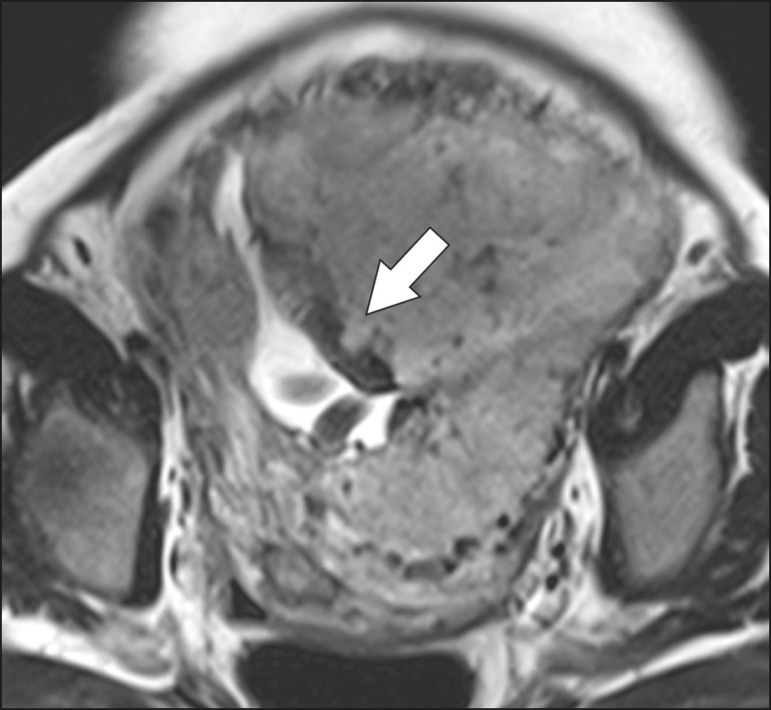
Sagittal T2-weighted MRI sequence showing placental heterogeneity, characterized by a diffuse signal and a dark intraplacental band (arrow).
